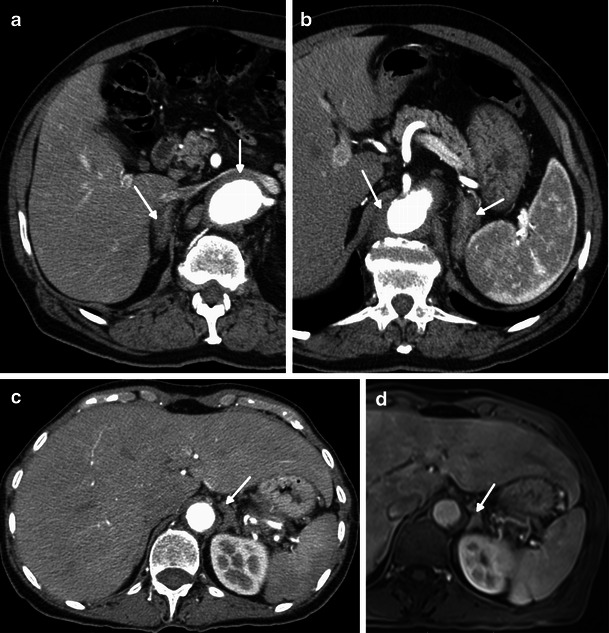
Axial CT-enhanced images in a 73-year-old male showing bilateral and diffuse thickening of the right (a) and left (b) adrenal glands (arrow). Periaortic concentric soft tissue infiltration is visible (arrow). Axial CT-enhanced (a) and axial T1 post-gadolinium (b) images in a 71-year-old female also showing diffuse thickening of the left adrenal gland (arrow)
